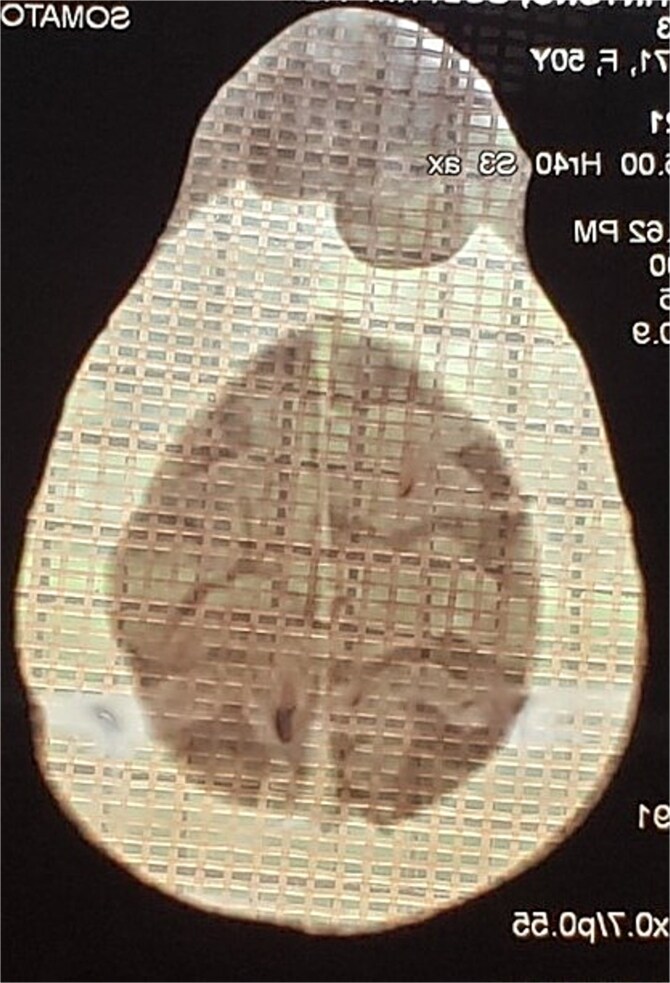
CT scan of the head demonstrating a frontal forehead mass with extension to the underlying skull.
